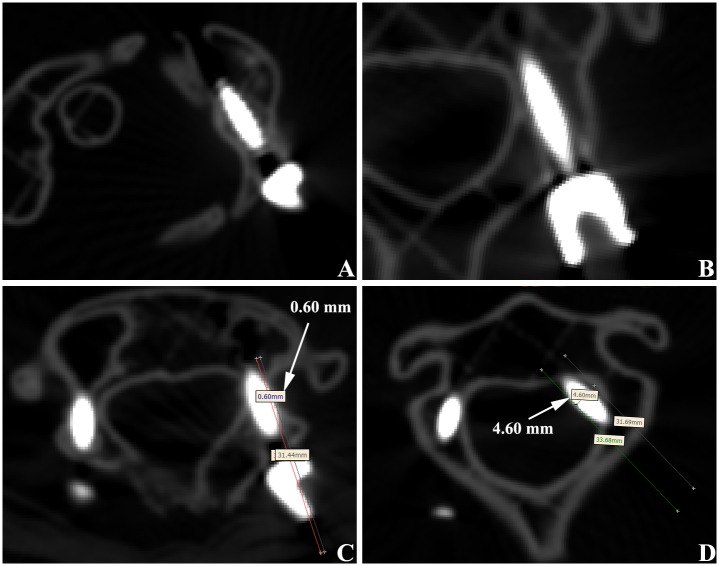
CT evaluation of pedicle screw fixation in upper cervical spine. (A) Atlas pedicle screw was totally in vertebral pedicle, which was considered as type I. (B) Axis vertebral pedicle screw was totally in vertebral pedicle, which was considered as type I. (C) Atlas pedicle screw broke lateral cortex of pedicle with a distance of 0.60 mm, which considered as type II. (D) Axis vertebral pedicle screw broke lateral cortex of pedicle with a distance of 4.60 mm, which was considered as type III.
